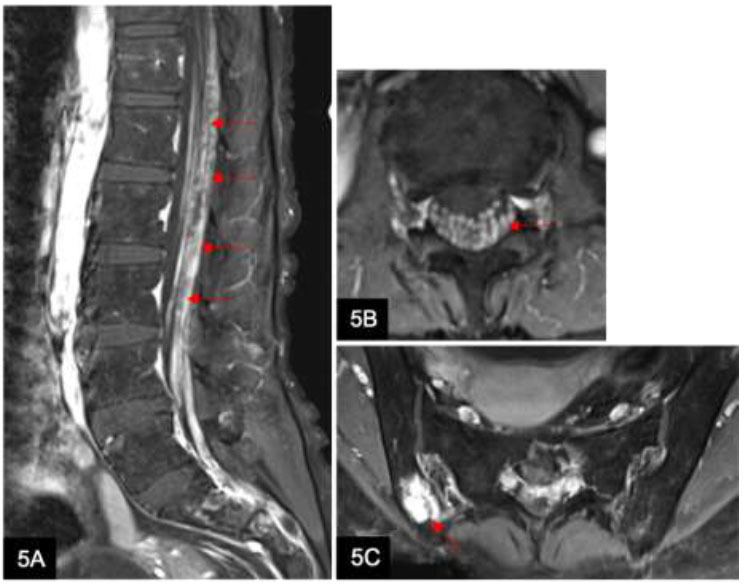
A 49-year-old woman with mantle cell lymphoma presents with bilateral lower limb weakness. Sagittal T1w post-contrast (A) and axial T1w post-contrast (B) MRI lumbar spine show extensive leptomeningeal enhancement along the cauda equina with a “sugar-coating” appearance (arrows). Axial T1w post-contrast (C) shows abnormal marrow infiltration (arrow) with enhancing foci in the pelvic bones. These are consistent with the leptomeningeal spread of the lymphoma in the spine.
